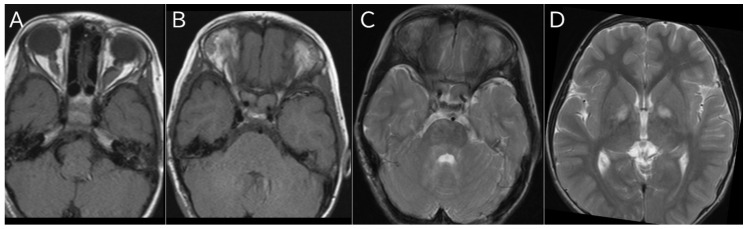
MRI imaging findings of NF1. (A,B) Bilateral optic gliomas in a 4-year-old girl; (A) T1-weighted image; (B) T2-weighted image. (C) T2-weighted images of UBOs in bilateral cerebellum of a 4-year-old girl. (D) T2-weighted image of UBOs in bilateral globus pallidus in a 7-year-old boy.
